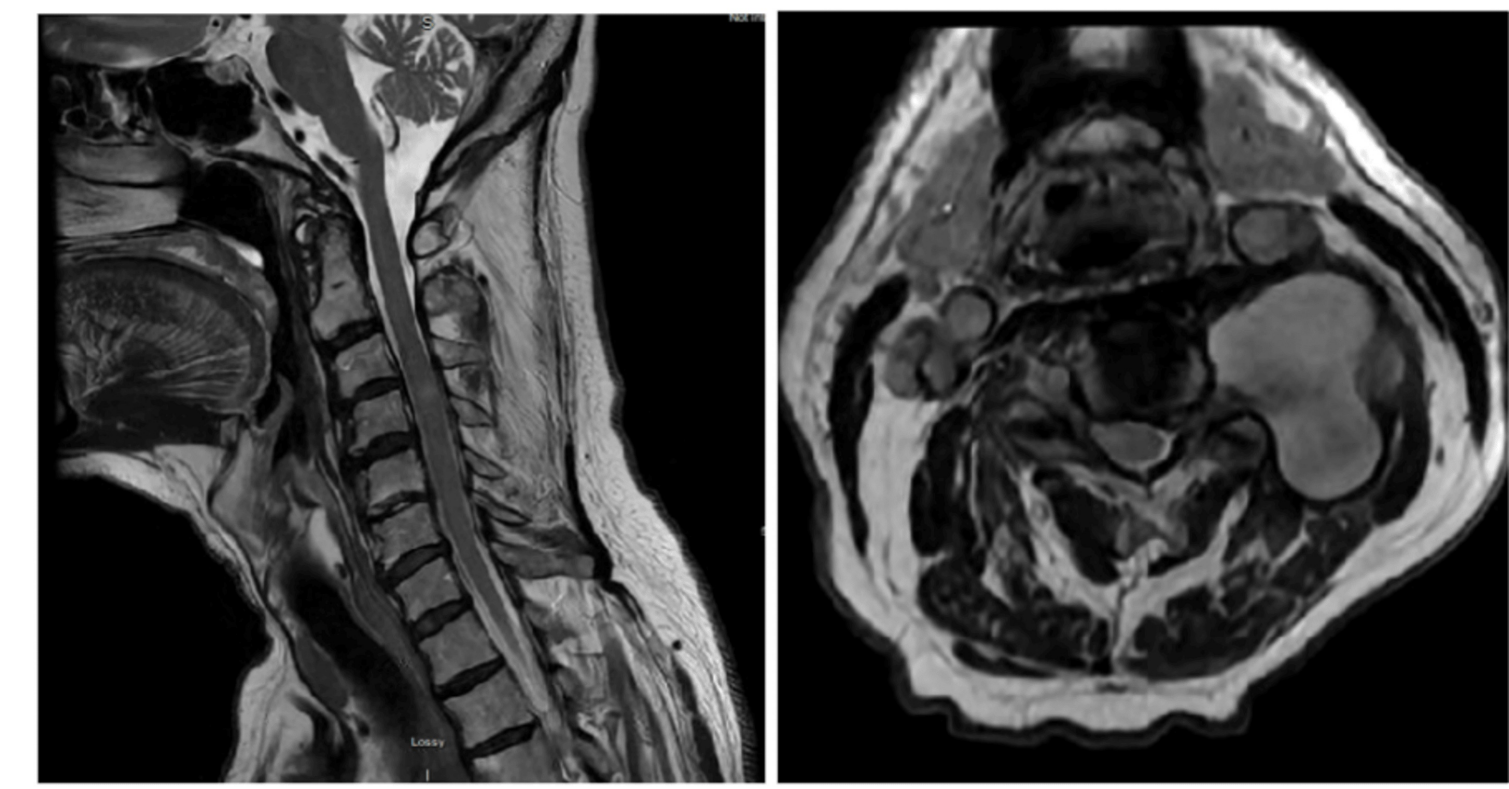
Magnetic Resonance Imaging (MRI) of cervical spine MRI of cervical spine reveals a lobular T2 hyperintense lesion adjacent to C4, measuring 4.1 x 3.1 x 4.1 cm, with heterogeneous T1 signal. The lesion extends towards the left vertebral artery. Notable findings include increased signal at C4, suggesting cord edema, and degenerative changes with varying degrees of central canal and foraminal stenosis at multiple cervical levels.
